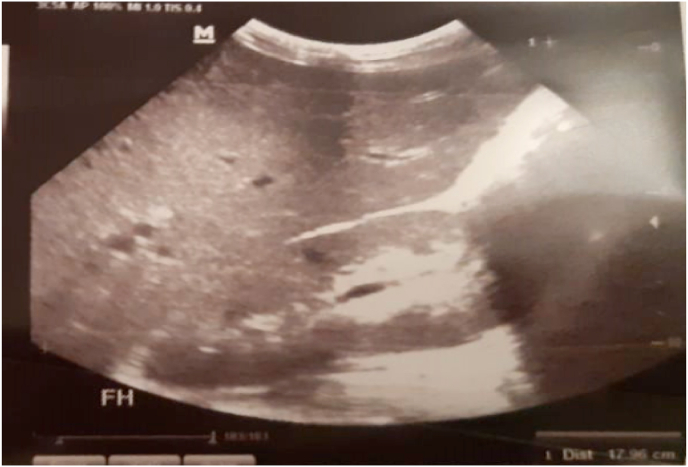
Abdominal ultrasound: showing hepatomegaly with regular contours and homogeneous structure.
